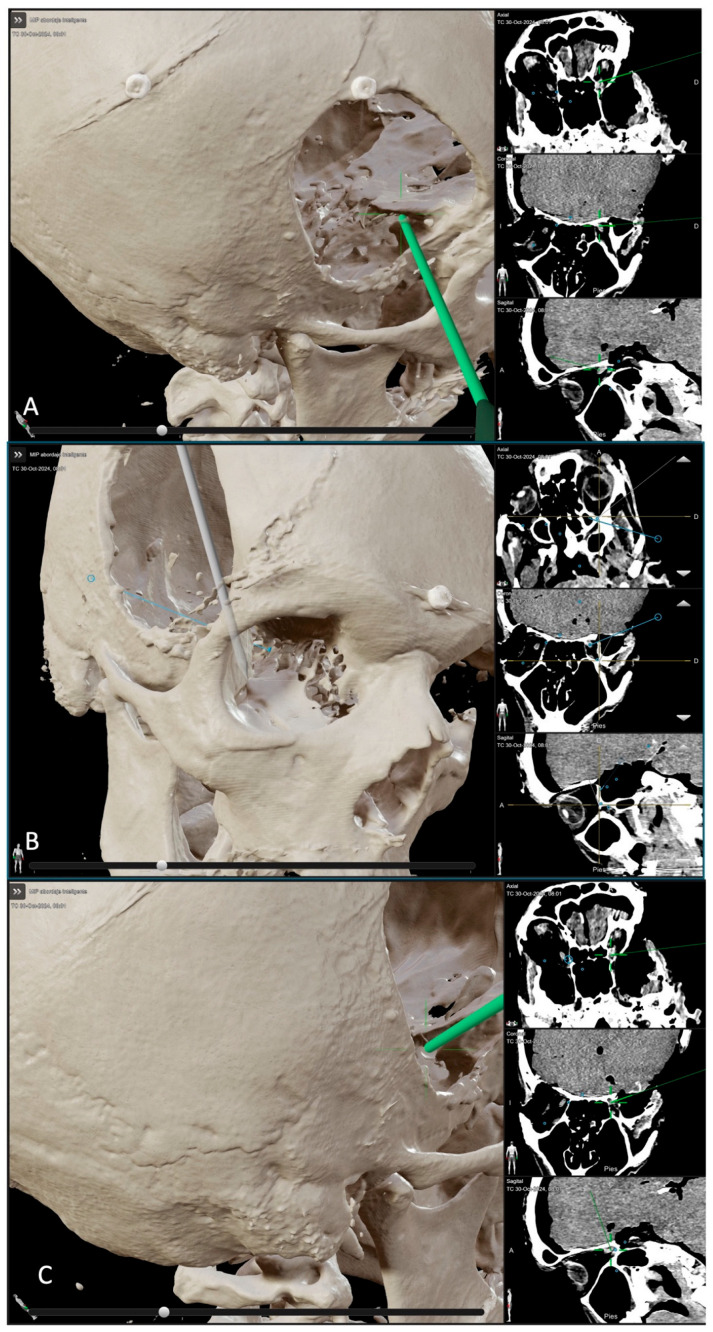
BrainLab^®^ CT reconstruction post right transcranial dissection. (A–C) Neuronavigation showing distances to SOF (A), IOF (B), and OC (C), relative to spheno-orbital meningiomas.
